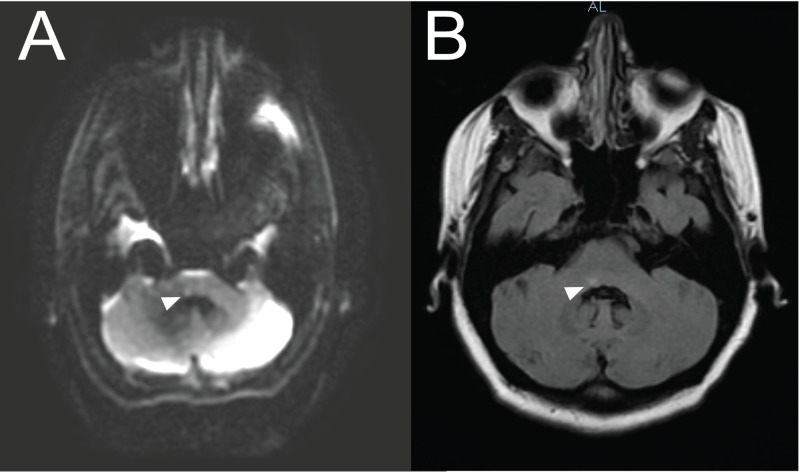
Brain MRI (A) Initial MRI (with contrast) showing increased focus on the diffusion-weighted imaging series in the dorsal aspect of the right pons at the floor of the fourth ventricle corresponding to the right facial colliculus, indicated by the arrowhead. (B) Second MRI performed on day 2 of admission showing an increased focus in the pons, indicated by the arrowhead.
